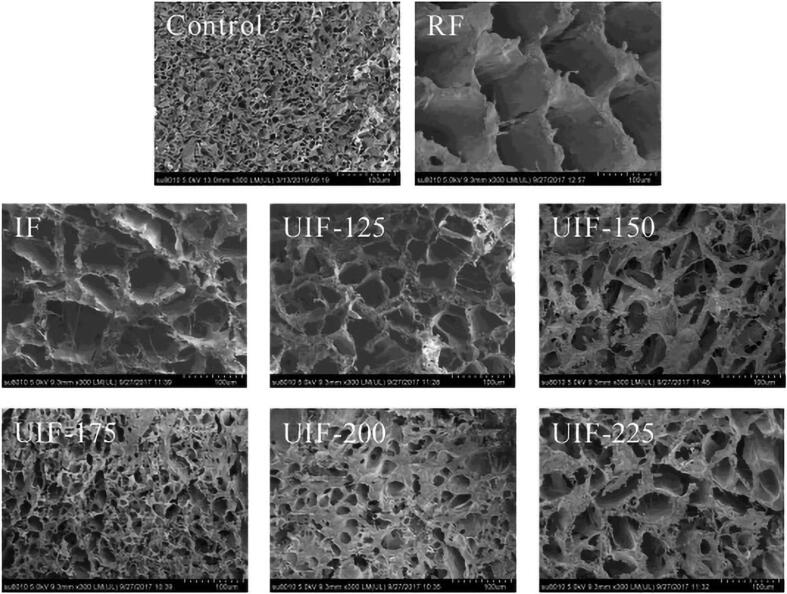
SEM images of carp muscles with different cryopreservation treatments (magnification: 300x). RF: refrigerator freezer; IF: immersion freezing; UIF: ultrasound immersion freezing at various ultrasound powers (125 W, 150 W, 175 W, 200 W, and 225 W) [9].
